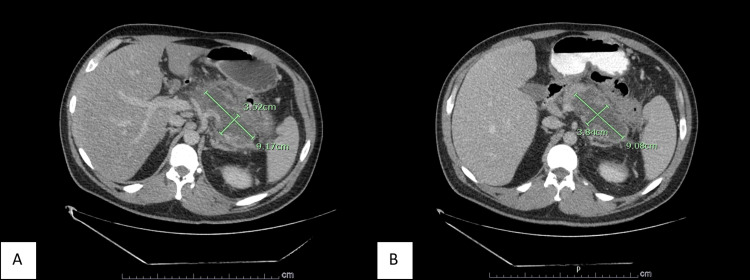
Transverse section CT scan of the abdomen/pelvis (A) Day 1: The pancreatic/peripancreatic inflammation is most prominent in the body and tail regions of the pancreas, with associated necrosis and pseudocyst formation at the pancreatic body and tail measuring 9.2 cm x 3.5 cm. (B) Day 5: Stable acute pancreatitis with a peripancreatic collection measuring 9.1 cm x 3.8 cm
